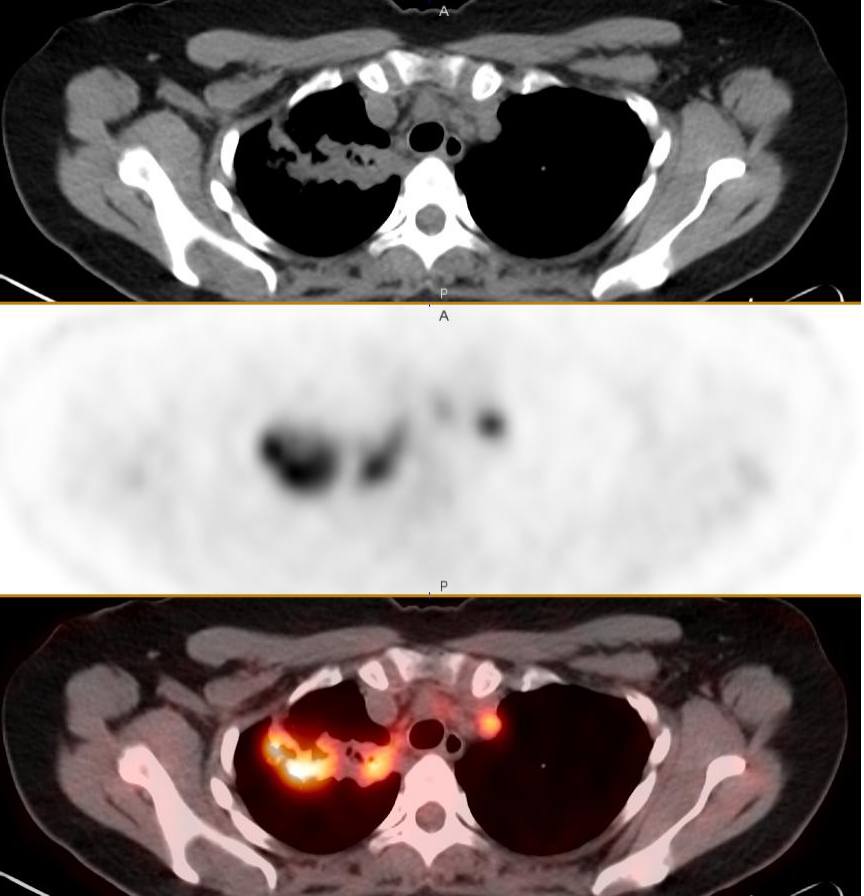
Axial series showing dense consolidation with areas of increased uptake in the right upper lobe and focal uptake in a contralateral mediastinal node: CT, positron emission tomography and fused PET/CT images.
